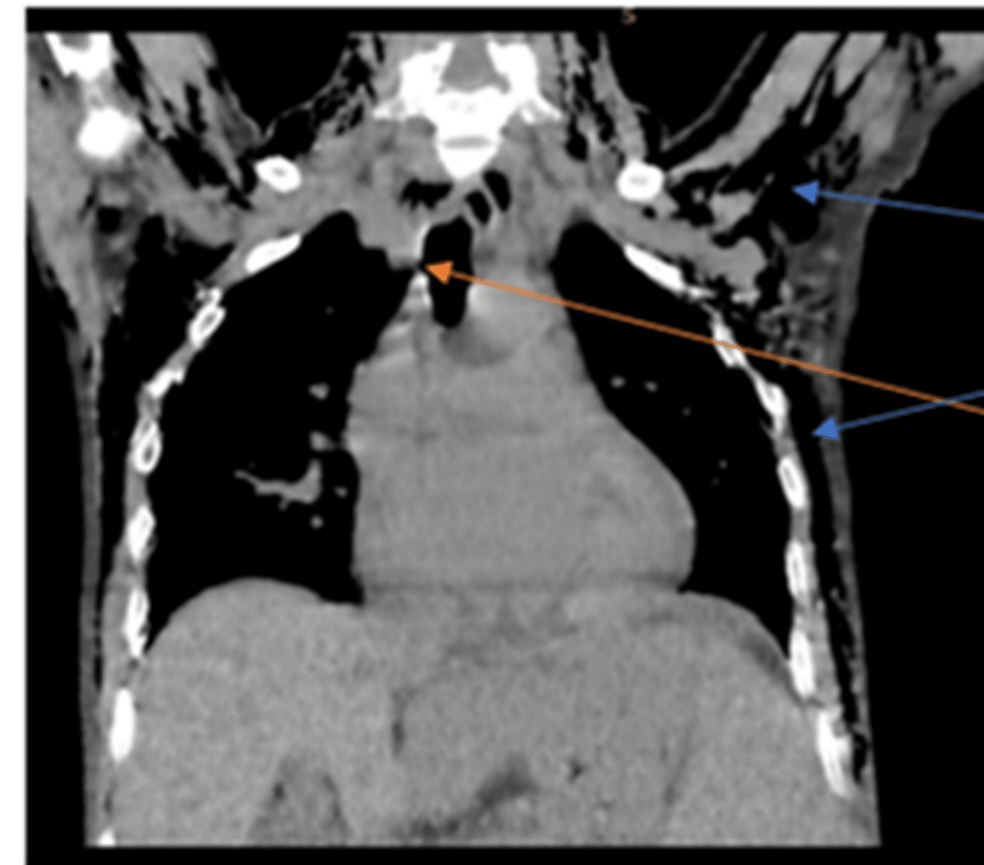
High-resolution computed tomography (HRCT) of the thorax (coronal view) showing the presence of tracheoesophageal fistula (red arrow) and subcutaneous emphysema (blue arrow)
